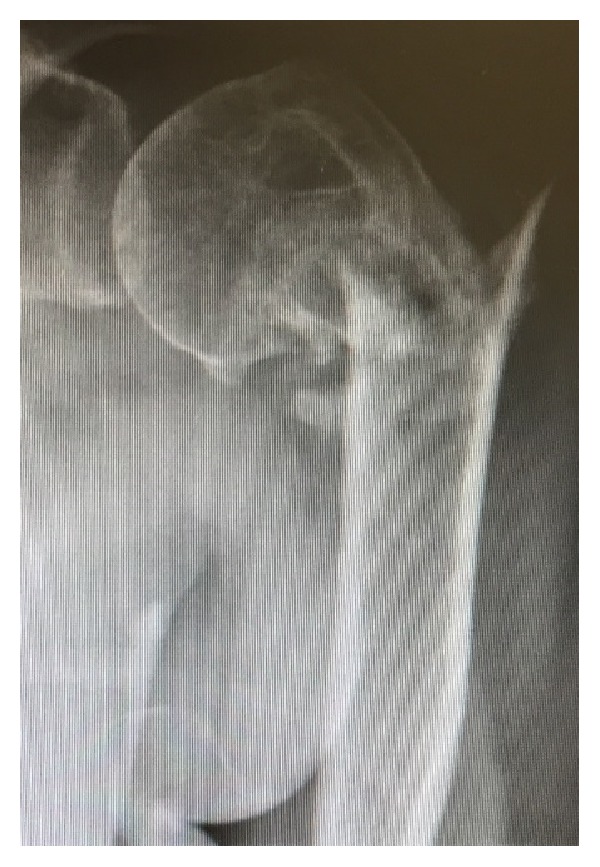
Preoperative radiograph depicting a two-part fracture of the proximal humerus. Note the varus malalignment of the head and lateral bone spike.
